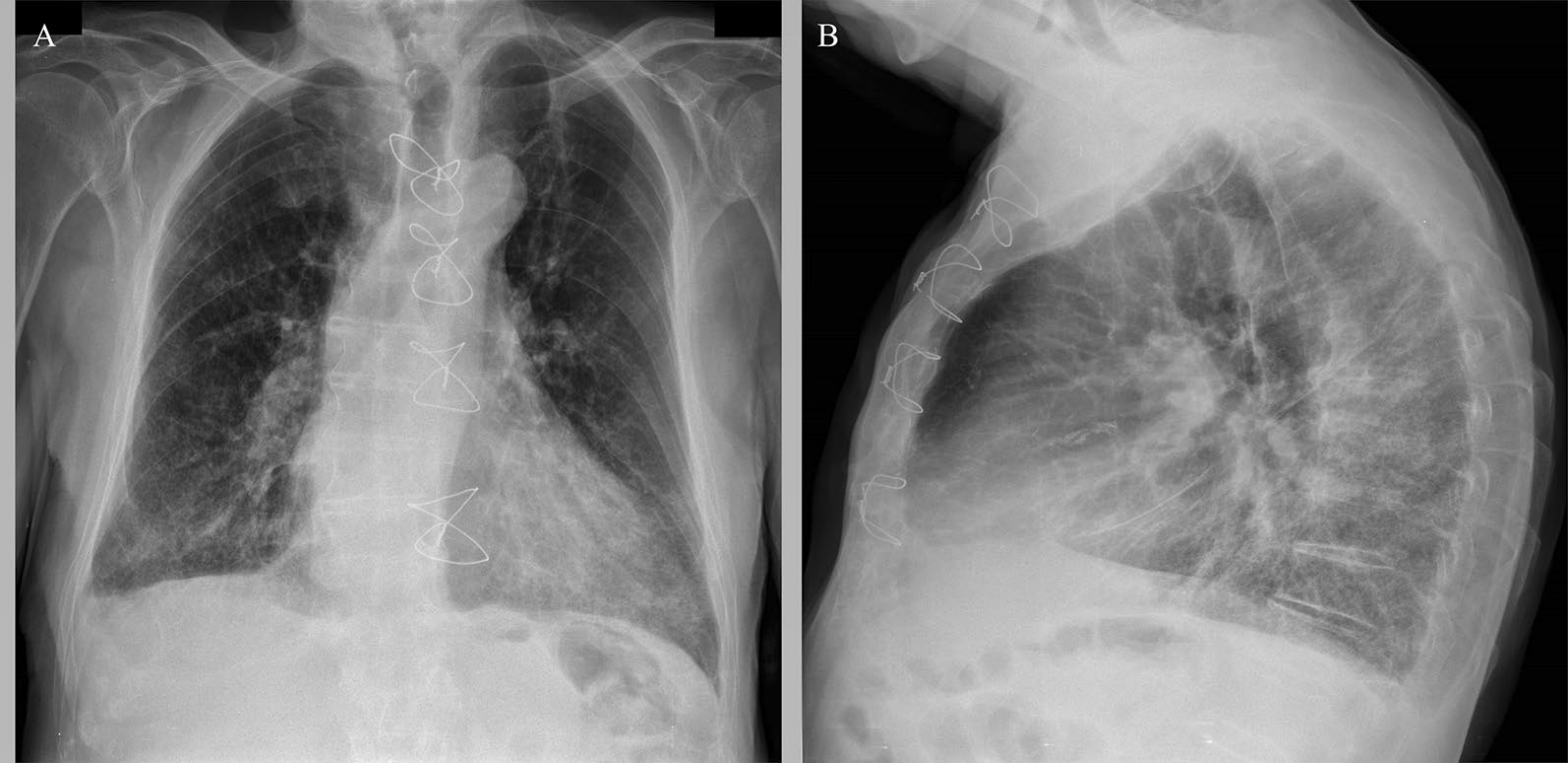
Chest X-ray images obtained upon admission to LCID. Chest X-ray findings included infiltrations in the lower fields of both lungs, cardiomegaly, Kerley B lines, upper lobe pulmonary venous congestion, right pleural effusion. Bilateral basal pneumonia and heart failure X-ray signs. A Posterior-anterior chest X-ray; B Lateral view chest X-ray
